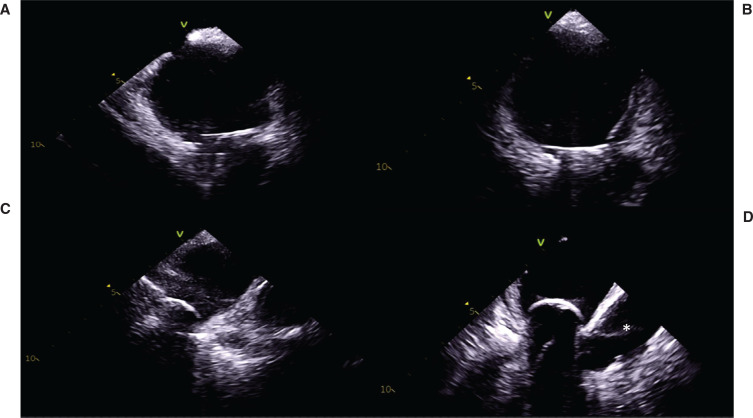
Proper cryoballoon positioning using intracardiac echocardiography in the (A) left superior, (B) left inferior, (C) right inferior, and (D) right superior pulmonary veins. Asterisk indicates the right pulmonary artery, which typically comes into view while visualizing the right superior pulmonary vein.
